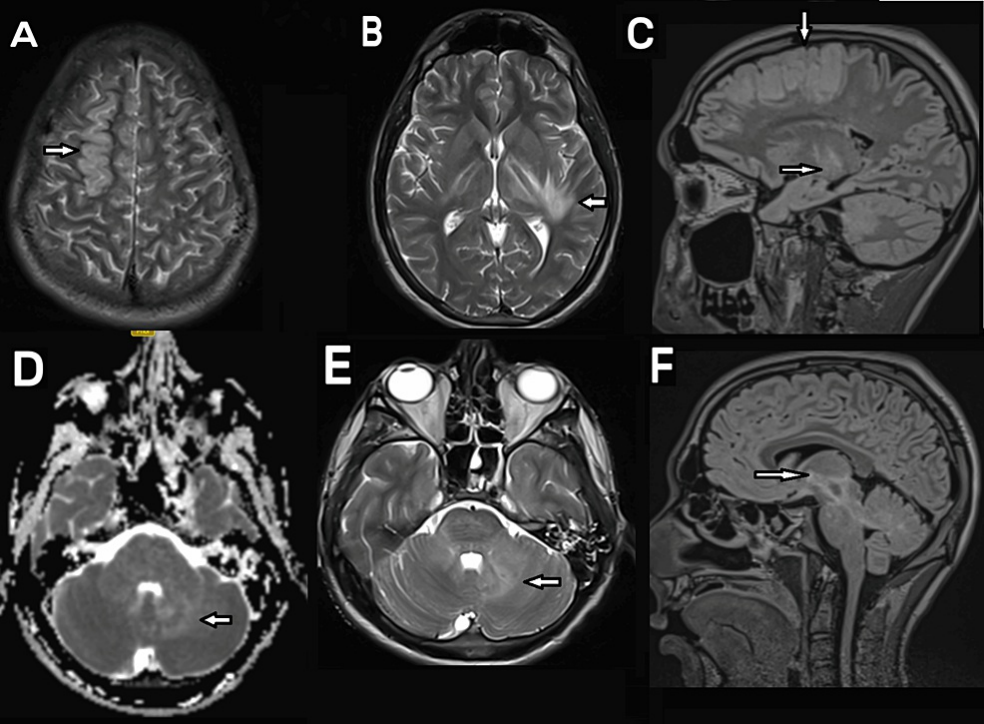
MRI with contrast with arrows demonstrating multifocal involvement of the right superior frontal gyrus (A and C), posterior limb of left internal capsule and subcortical U-fibres in the adjacent temporal lobe (B and C), cerebellum (D and E), and brainstem (F)
